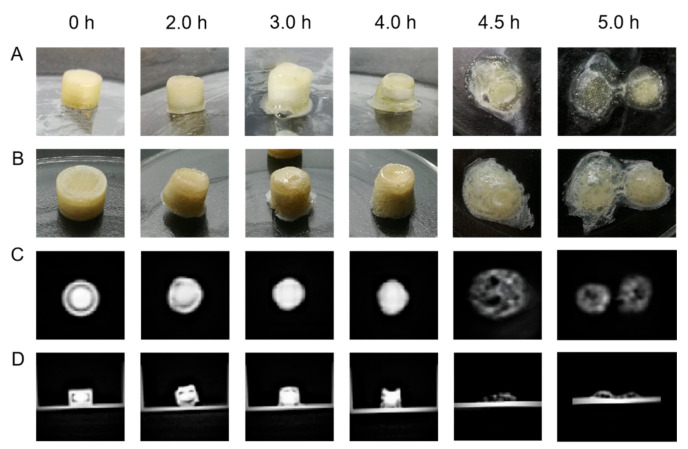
The changes of the 3D-printed tablets during dissolution: pictures of the tablet before (A) and after (B) freeze-drying process, CT images of cross section (C) and vertical section (D) of the tablet.
